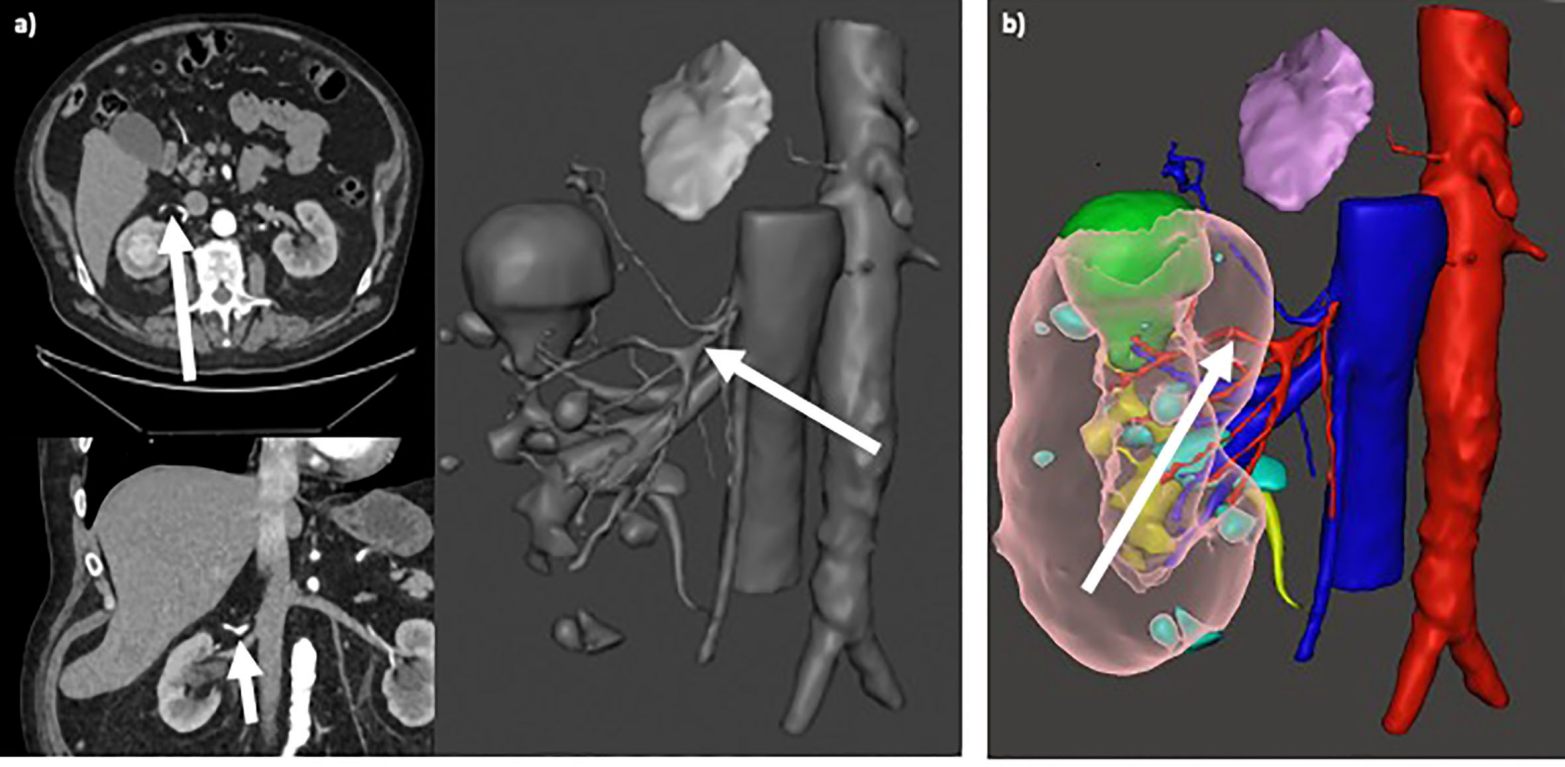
(A) Selective clamping of primary arterial branching (arrows in axial/coronal views and in 3D rendering), resulting from preoperative planning based on standard 2D CT imaging; (B) Super-selective clamping of tertiary arterial branching (arrow), resulting from preoperative planning based on 3D model.
